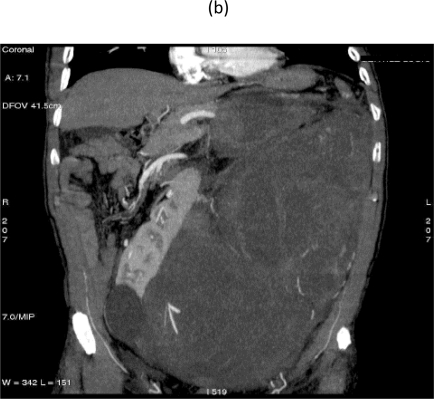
Retroperitoneal liposarcoma, as visualized in contrast-enhanced multidetector computed tomography (MDCT) of abdomen and pelvis. Multiplanar reformations (MPR) show the whole tumour extension, measuring 25 × 13 cm in the transverse plane (a) and 36 cm in the coronal plane (b).
